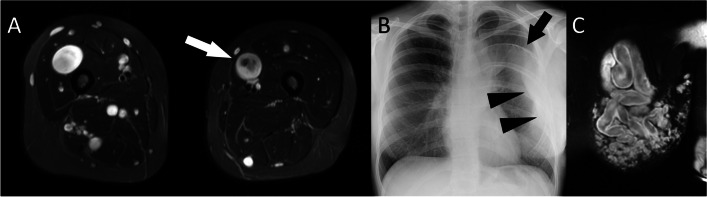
A Axial T2 fat-saturated MR image through both thighs in a patient with NF1 demonstrated multiple neurofibromas characterised by hyperintense signal in the periphery with hypointense signal centrally. B A PA chest radiograph in a patient with a plexiform neurofibroma demonstrates a soft tissue mass (arrowheads) and a scalloping of the left 5th rib. C A coronal T2W fat-saturated MR image through the left chest wall demonstrates a complex tubular mass with signal characteristics typical of a neurofibroma. The shape of the left 5th rib may be the result of focal dysplasia rather than a direct pressure effect
